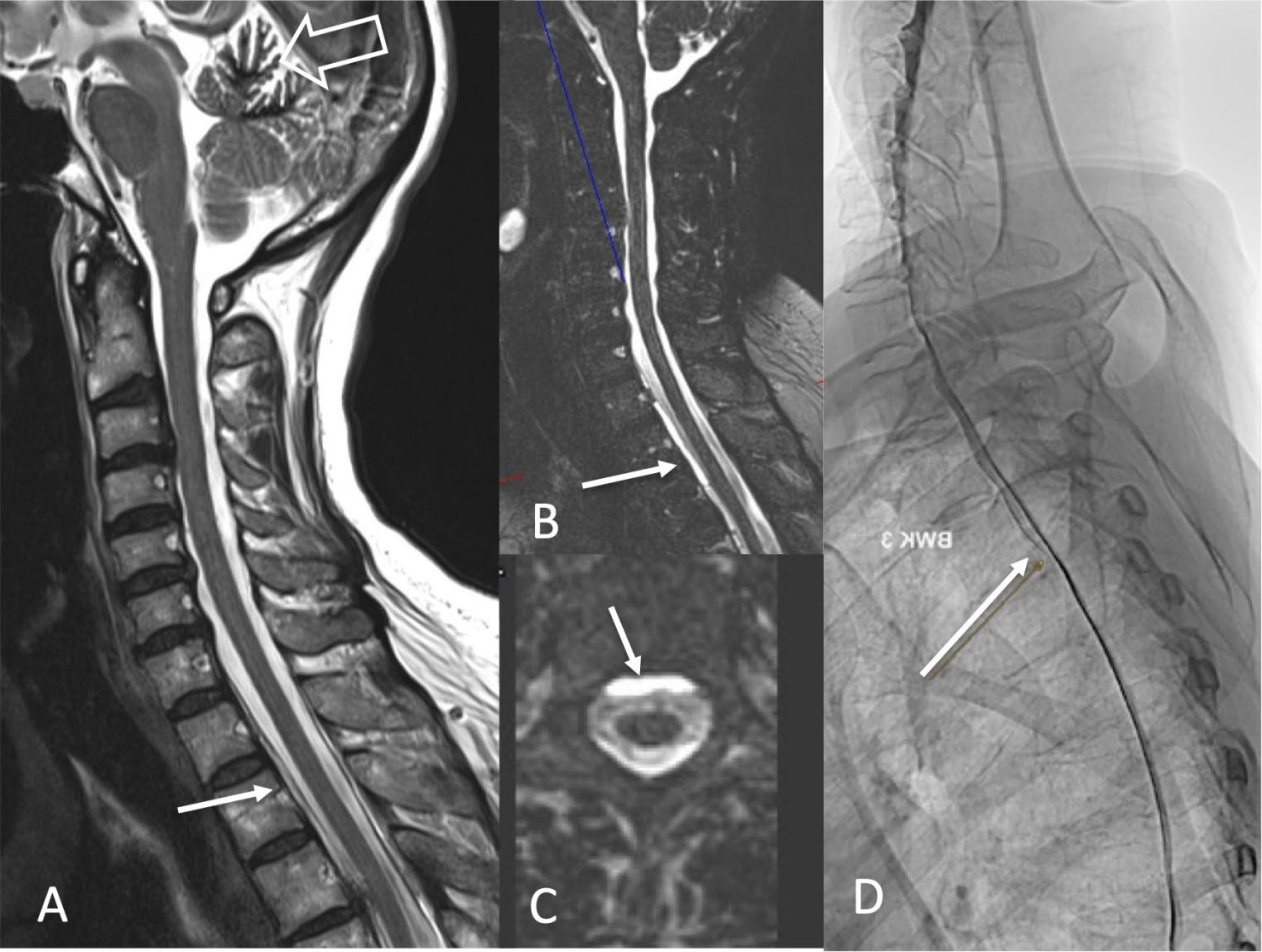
A 54-year-old woman complained of headache, head pressure ear ringing, blurry vision, gait disorder, and cognitive “slowing”. Sagittal T2-weighted MRI of the cervical spine shows infratentorial hemosiderosis with hemosiderin in the foliae of the upper vermis (A: hollow arrow) and spinal longitudinal epidural fluid (SLEC) (A: arrow). A heavily T2-weighted fat-suppressed SPACE sequence allows the calculation of axial images with the majority of the epidural fluid ventral to the spinal cord (B, C: arrow). Digital subtraction myelography with a high temporal resolution (1 frame/ second) was needed to capture the time point of egress of contrast from the subarachnoid space (D: arrow) – here, a non-subtracted image is displayed
